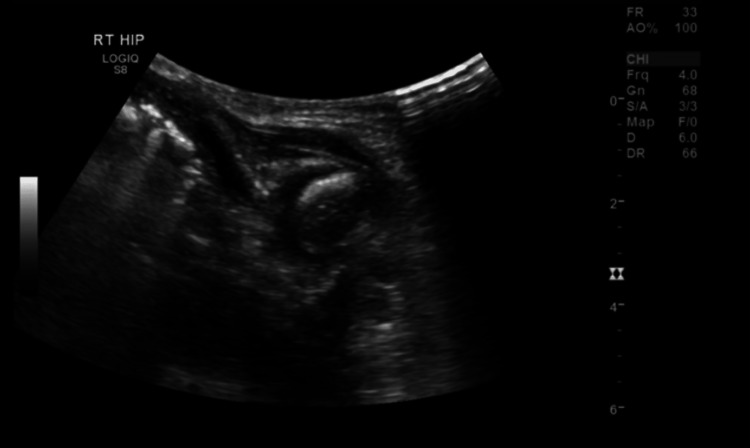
The ultrasound of the right hip showed moderate effusion.
